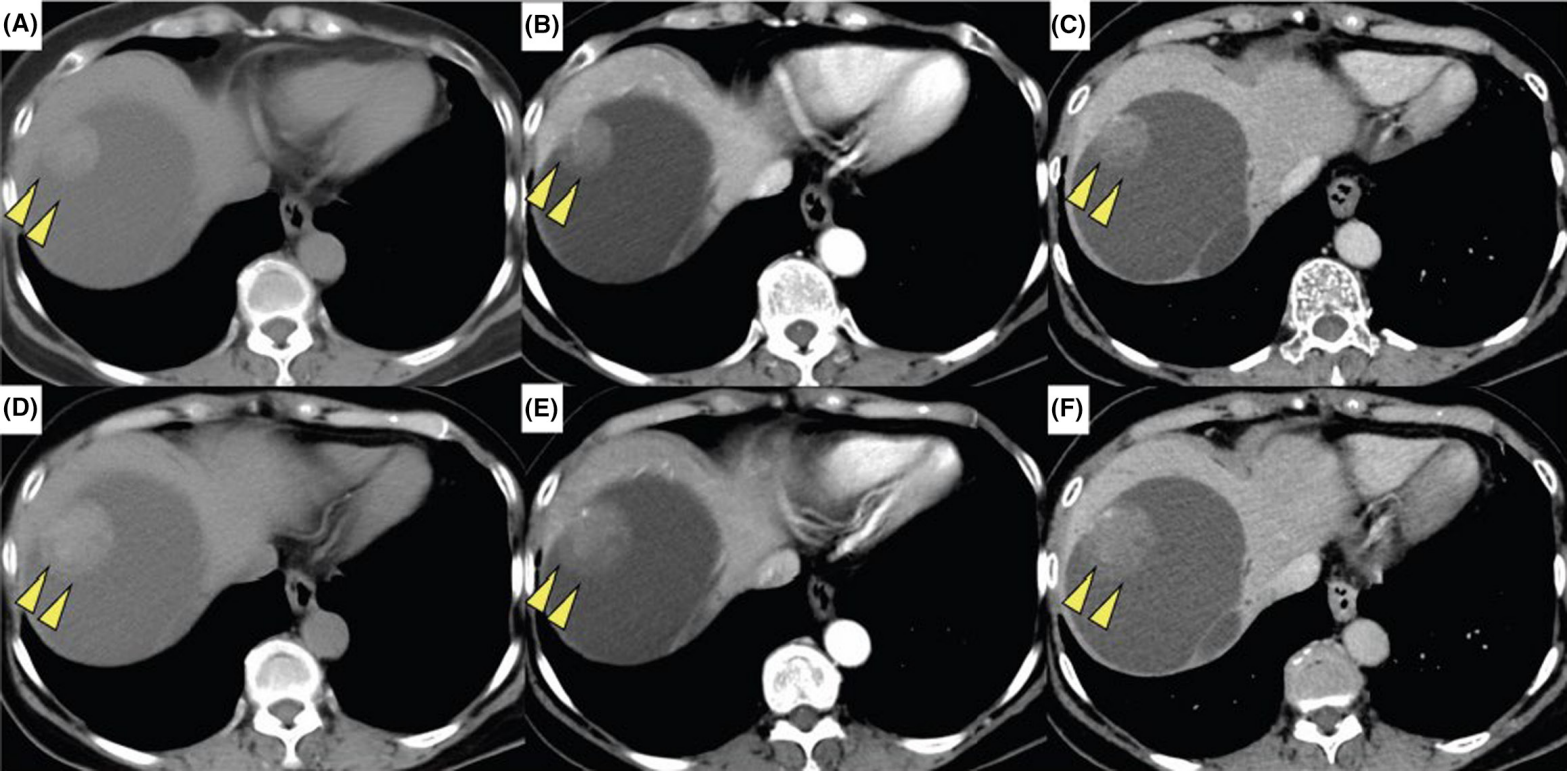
Preoperative computed tomography findings. Computed tomography conducted 4 months preoperatively (A–C) and computed tomography conducted 1 month preoperatively (D–F) reveal a 1.5‐fold increase in tumor size during the 3‐month period. The nodule within the liver cyst has a slightly higher density than the liver parenchyma (A, D), and a punctate high‐density area inside the nodule in the arterial phase (B, E) and delayed phase (C, F).
